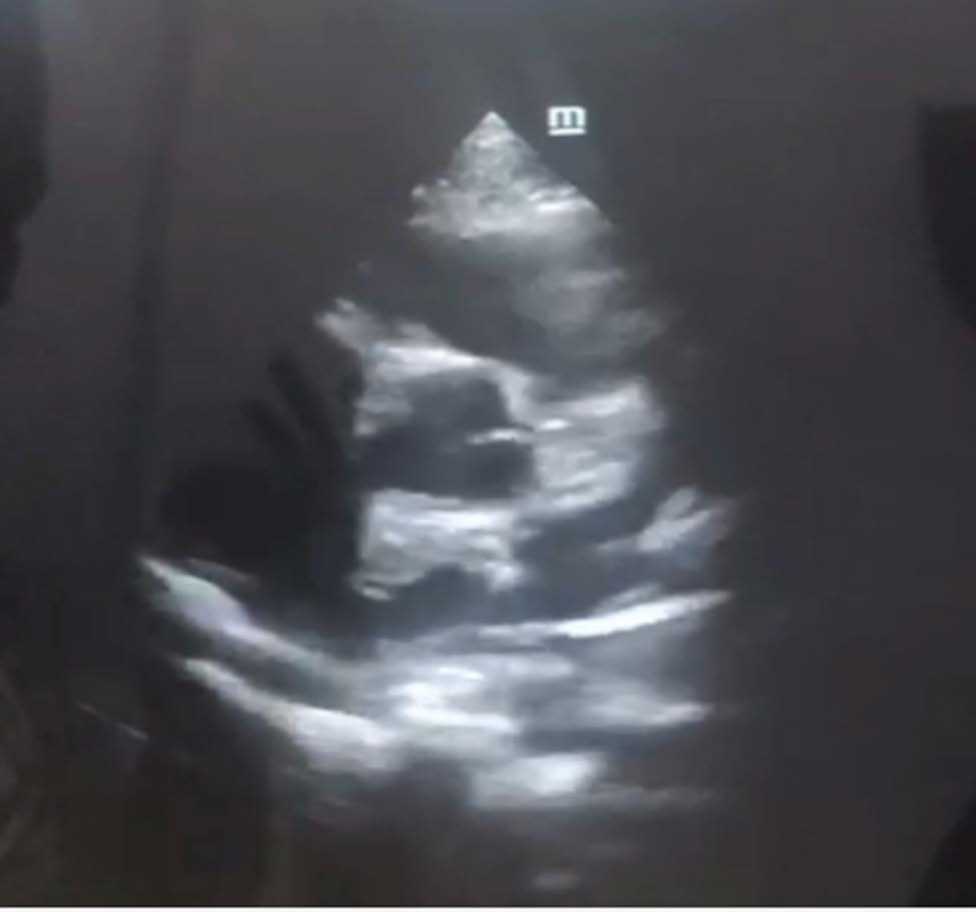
Echocardiographic image showing large embolus in the main pulmonary artery.
